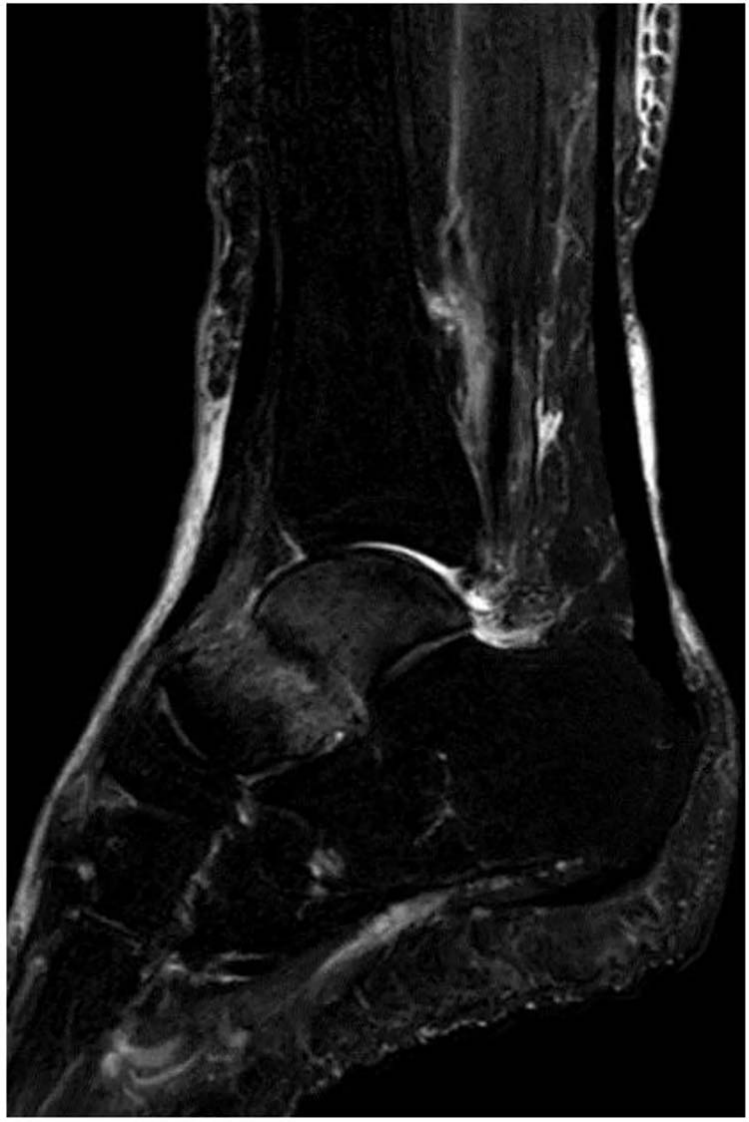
MRI revealed a low signal linear line in the talar neck and a bone marrow edema around the line on a fat-suppressed T2-weighted (FS-T2) image.
